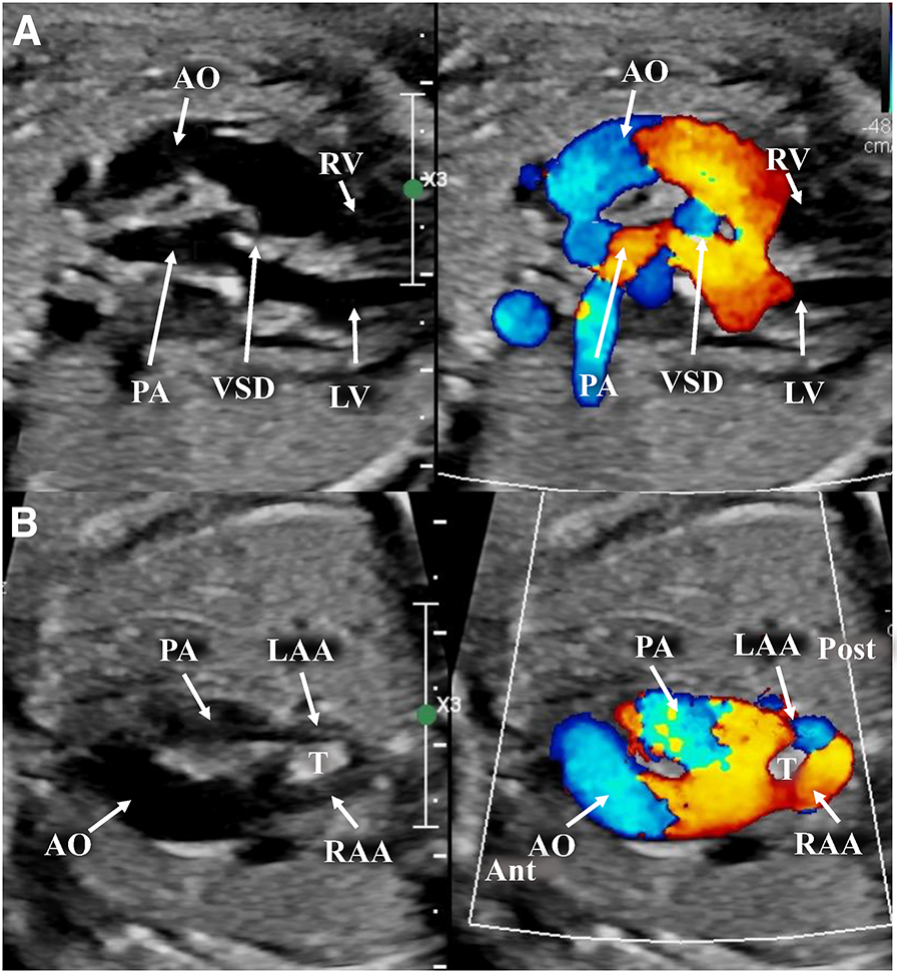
Images taken from a fetal transthoracic echocardiogram. (A) A long-axis view, with a color Doppler, where it is possible to observe the D-transposition with the anterior aorta and posterior pulmonary artery; the ventricular septal defect, with a dysplastic pulmonary valve. (B) An off-axis view at the level of the aortic arch, with a color Doppler, showing the anterior aorta with its complete vascular ring surrounding the trachea, consisting of a double aortic arch, and the posterior pulmonary artery. Ant, anterior; LAA, left aortic arch; LV, left ventricle; Post, posterior; RV, right ventricle; T, trachea.
